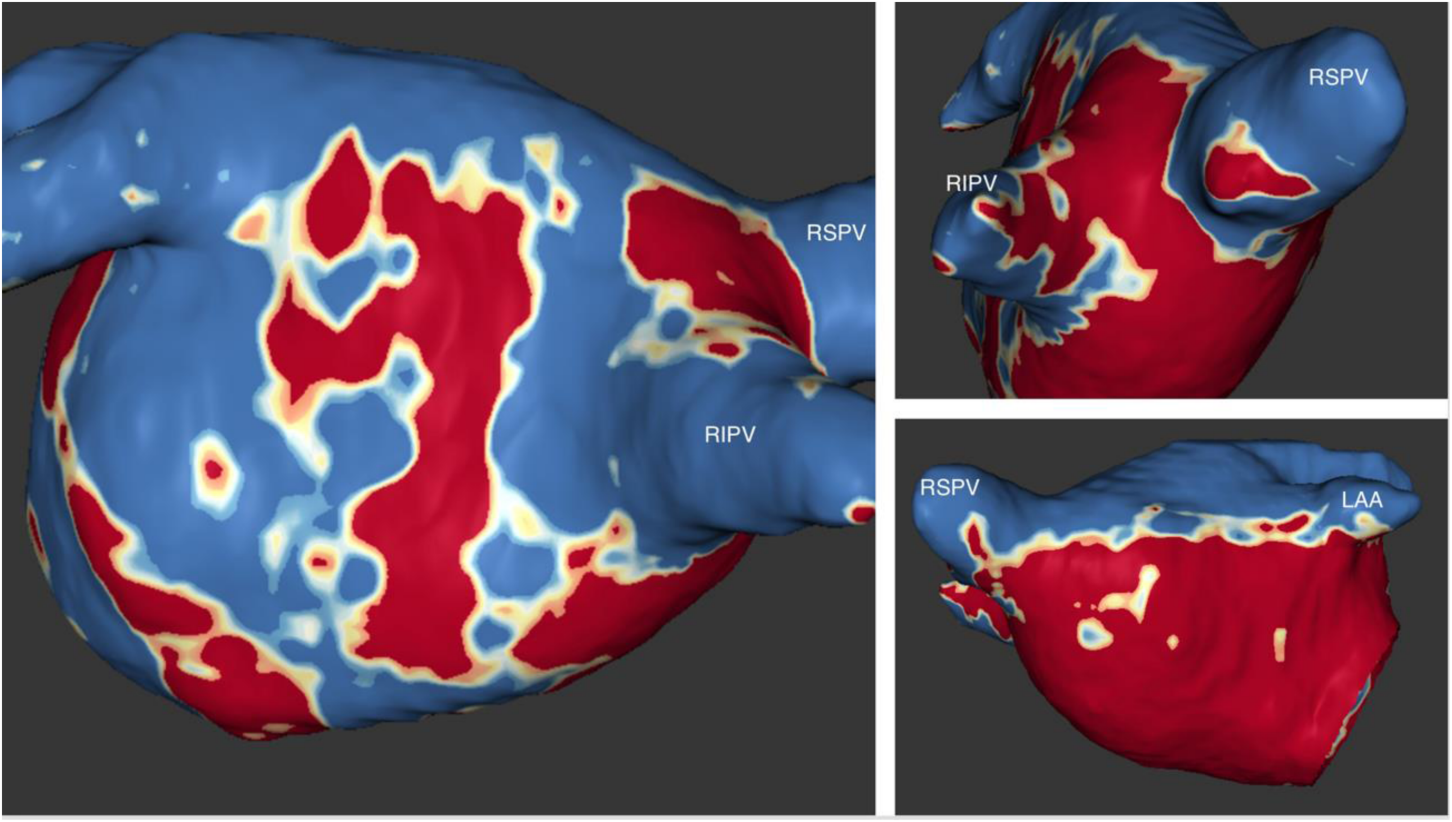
Images from late gadolinium enhancement (LGE) MRI. Fibrosis assessment with the ADAS 3 D software. The three-dimensional LA model from MRI shows extensive areas of fibrosis (dense scar) involving the posterior wall and the anterior and septal walls. LAA, left atrial appendage; RSVP, right superior pulmonary vein; RIPV, right inferior pulmonary vein.
